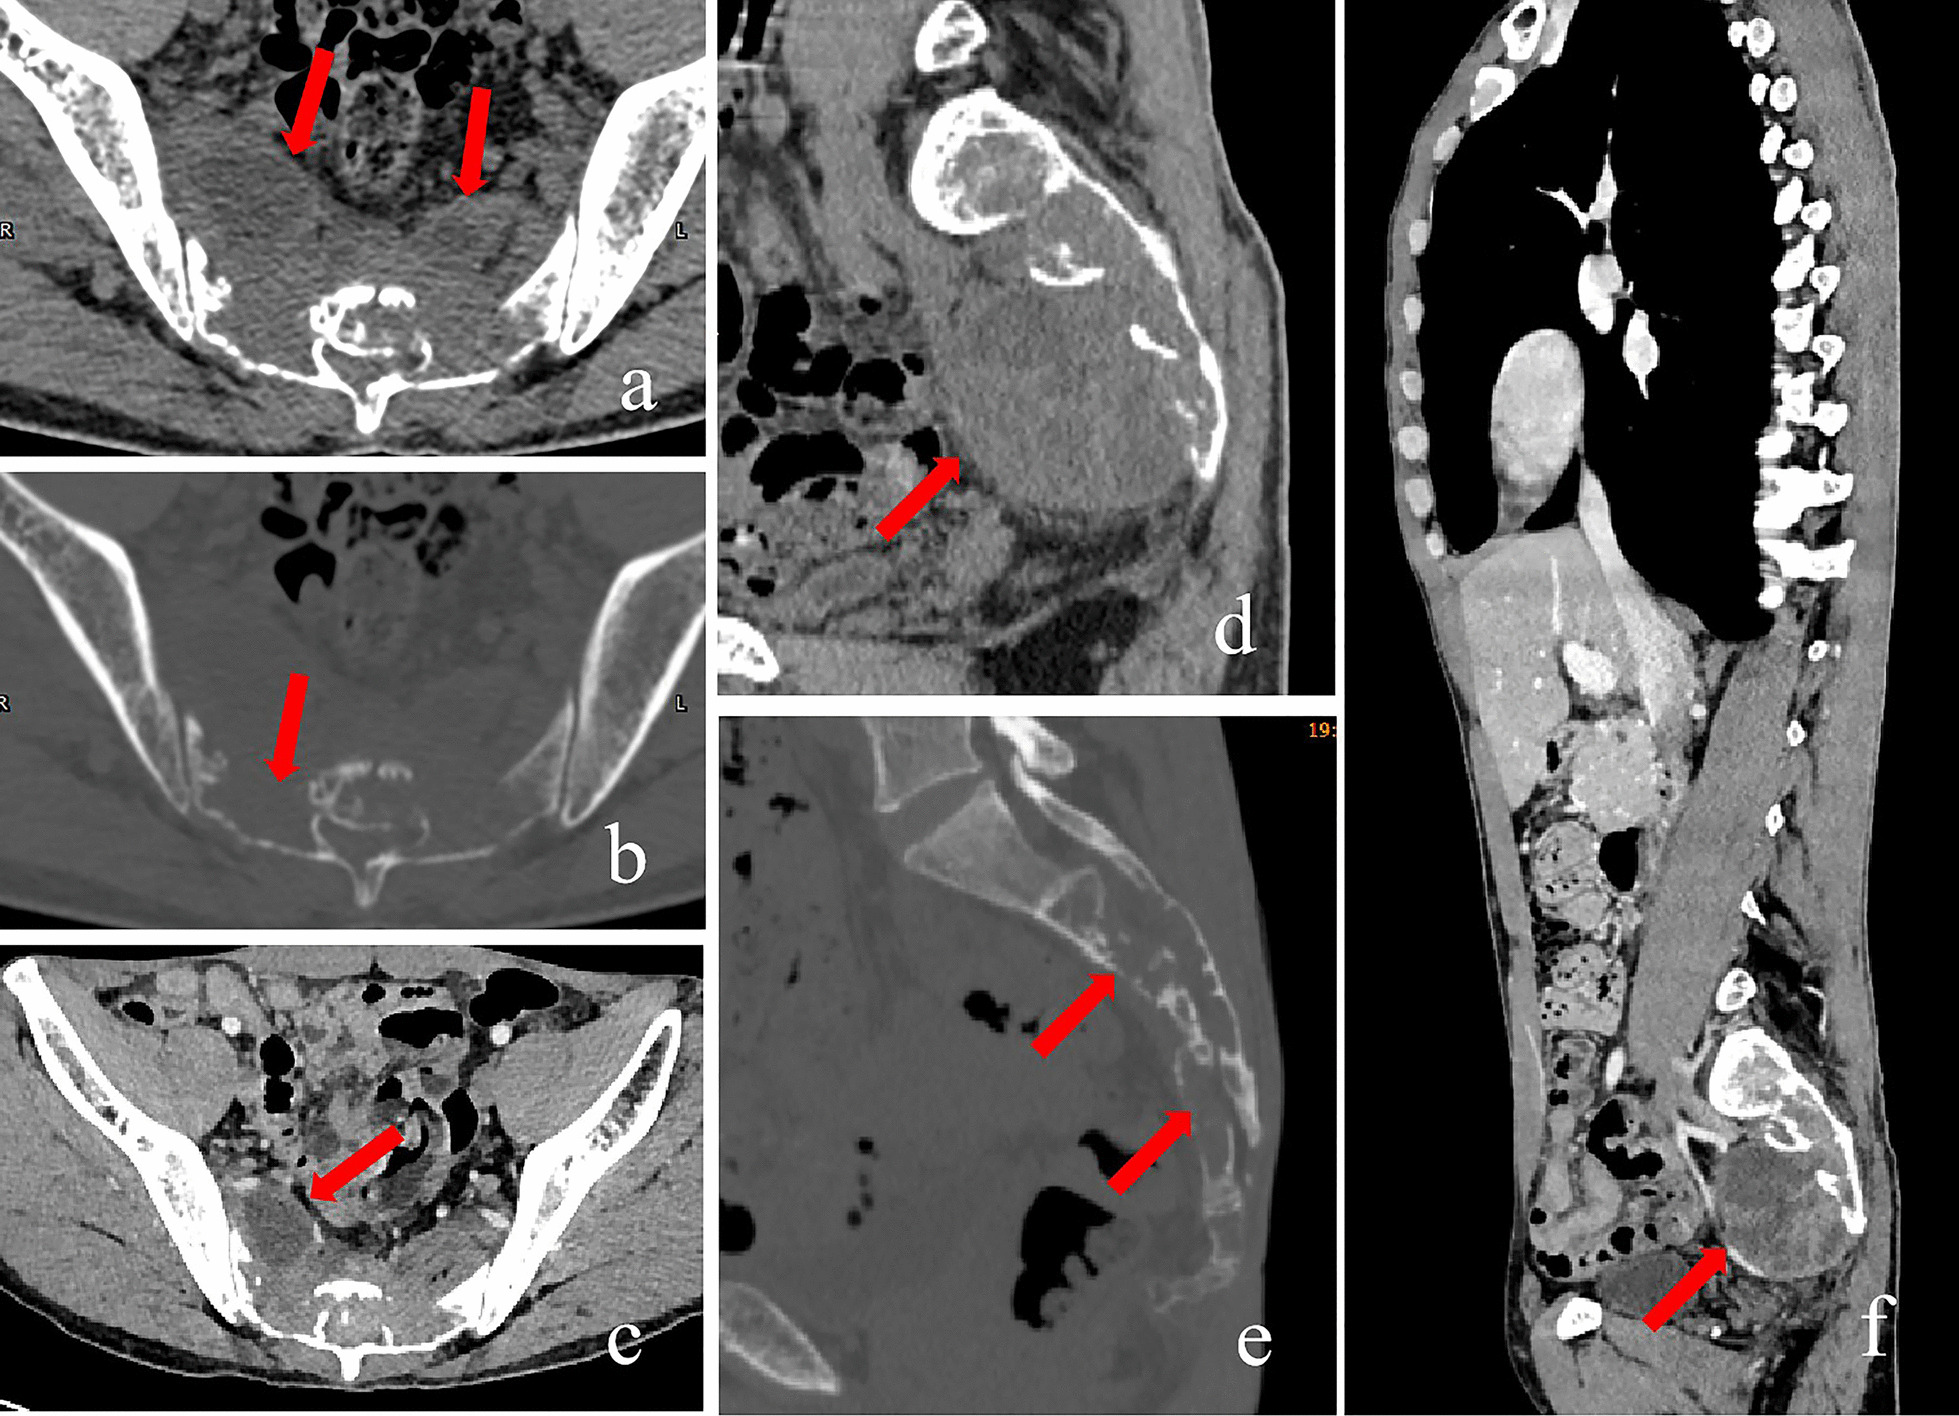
Preoperative computed tomography (CT) (sacrum). Non-enhanced CT scan (a transversal soft tissue window; b transversal bone window; d sagittal soft tissue window; e sagittal bone window): osteolytic lesions surrounded by cystic low-density soft-tissue masses of unequal-size, largest in anterior-right (red arrows). Contrast-enhanced CT (c transversal; f sagittal): soft-tissue masses with heterogeneous ring enhancement and unremarkable central enhancement (red arrows)
